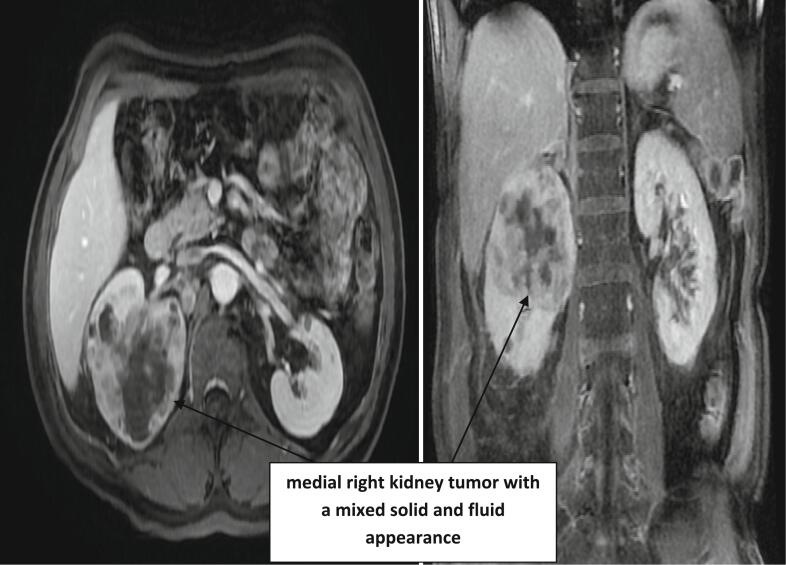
CT image of a right kidney tumor.
